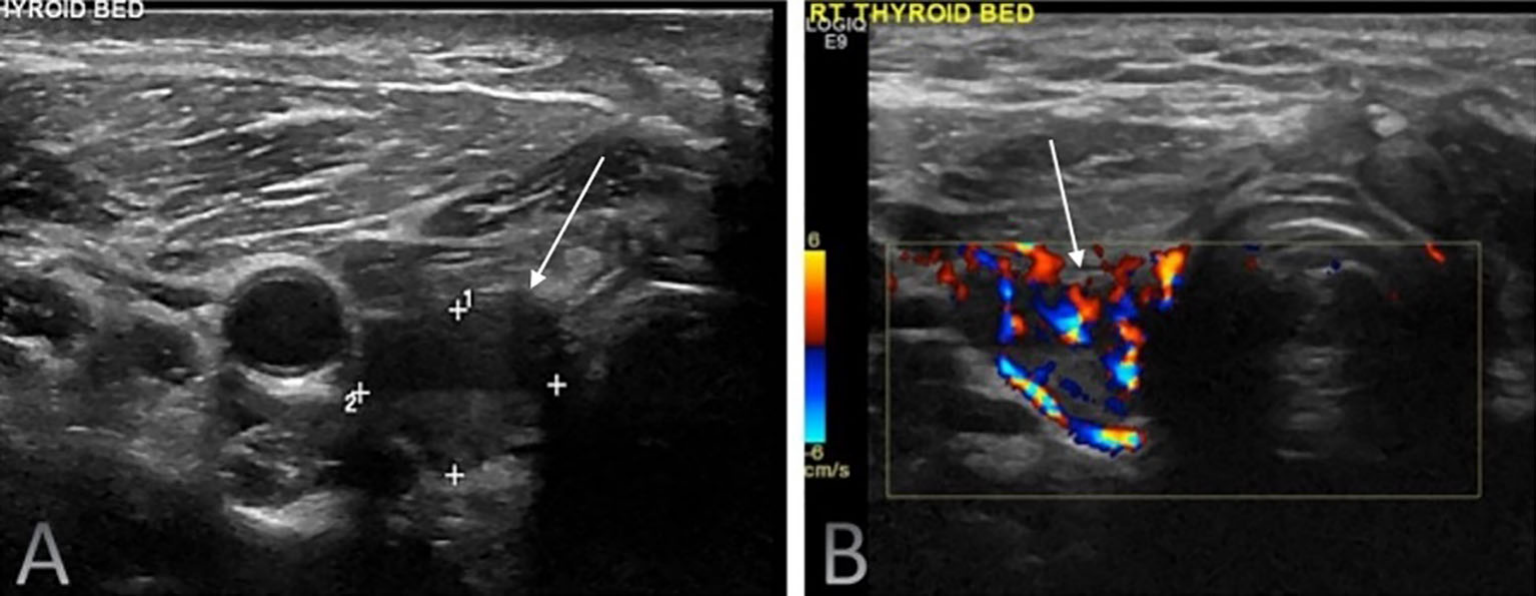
Routine surveillance duplex ultrasound of the right thyroid bed in a 41-year-old male with differentiated thyroid carcinoma (DTC) following total thyroidectomy and radioactive iodine therapy. The imaging demonstrated a highly suspicious solid hypoechoic nodule (white solid arrows) with loss of normal fascial planes on greyscale ultrasound (A) with internal vascularity seen on colour doppler (B). The serum Tg level was 8.6 ng/mL. Fine-needle aspiration cytology confirmed recurrent papillary thyroid carcinoma.
